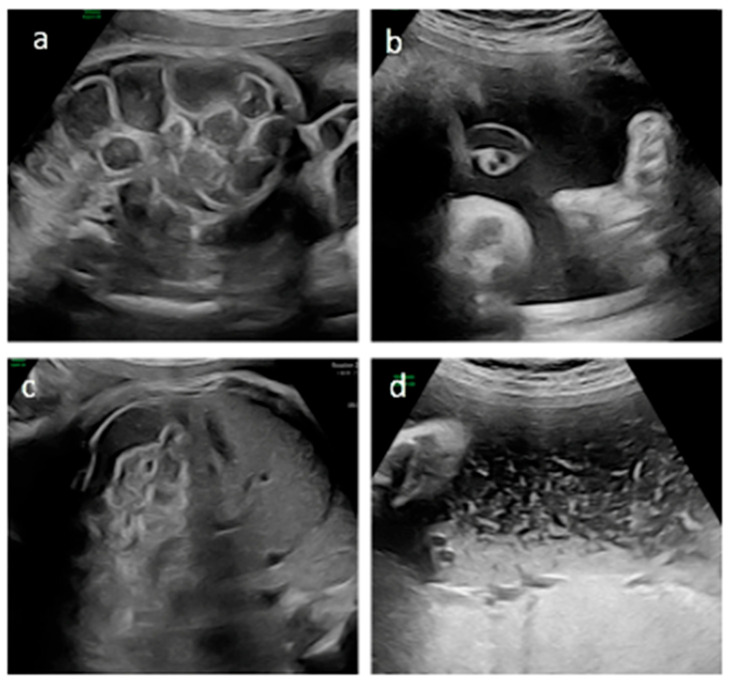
Ultrasound findings of the bowel and amniotic fluid before (a,b) and after (c,d) treatment with ETI. At 33 weeks’ gestation, diffuse bowel dilation with increased wall thickness (1.7 mm) and poorly echogenic amniotic fluid are observed (a,b). At 36 weeks’ gestation, reduced bowel caliber with persistent wall thickening and markedly echogenic amniotic fluid consistent with meconium are observed (c,d).
